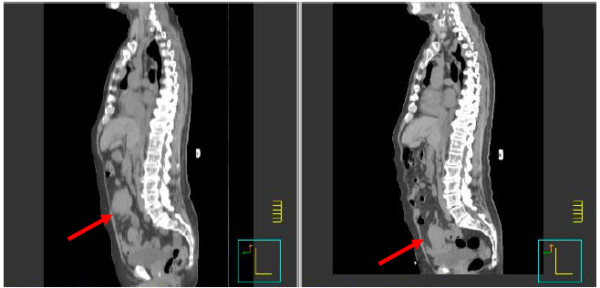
Sagittal treatment-planning computed tomography scans obtained five days apart showing the abdominal tumor in two distinctly different locations.
